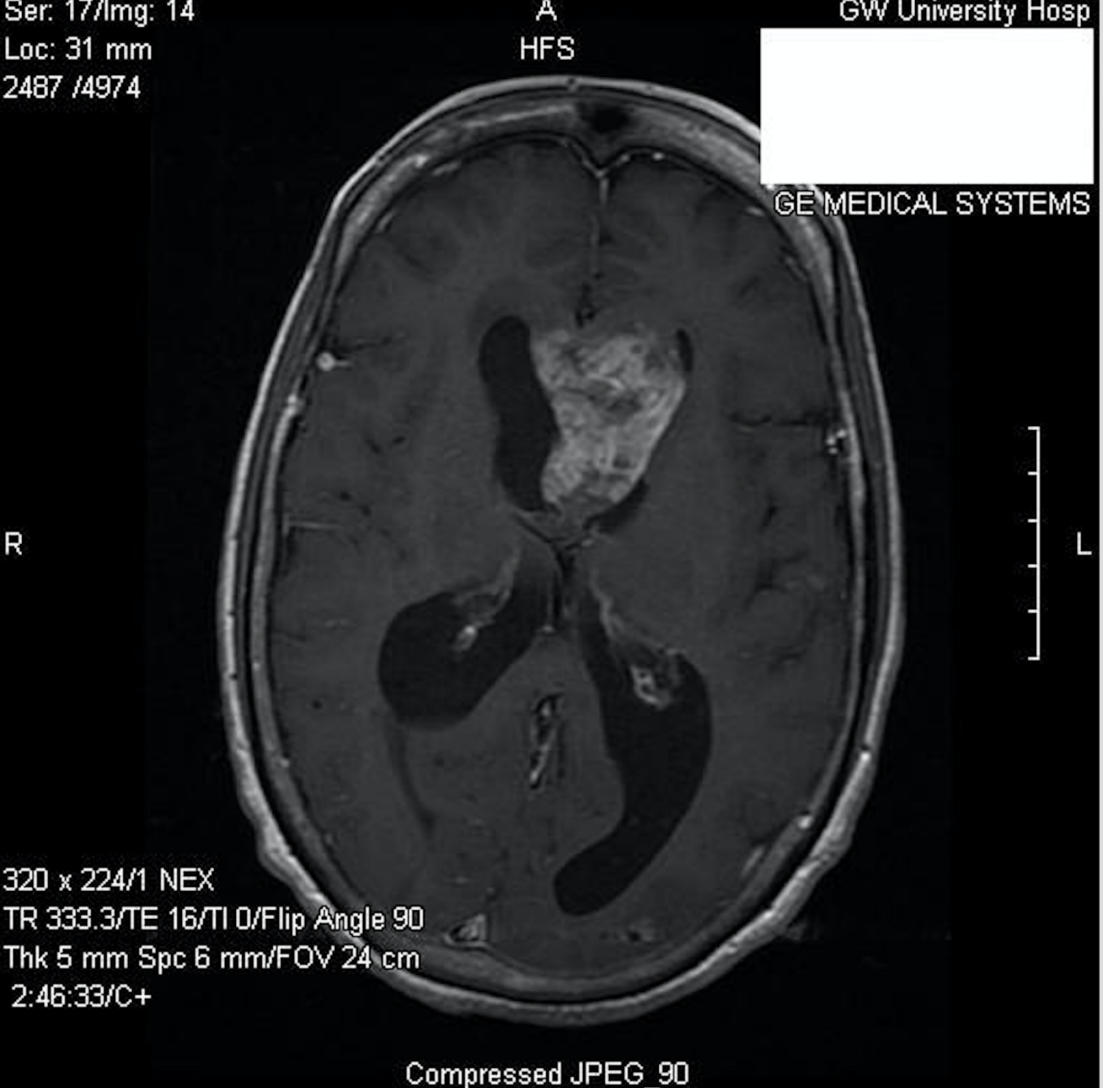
Preoperative axial post-contrast T1-weighted MR image MR: magnetic resonance
